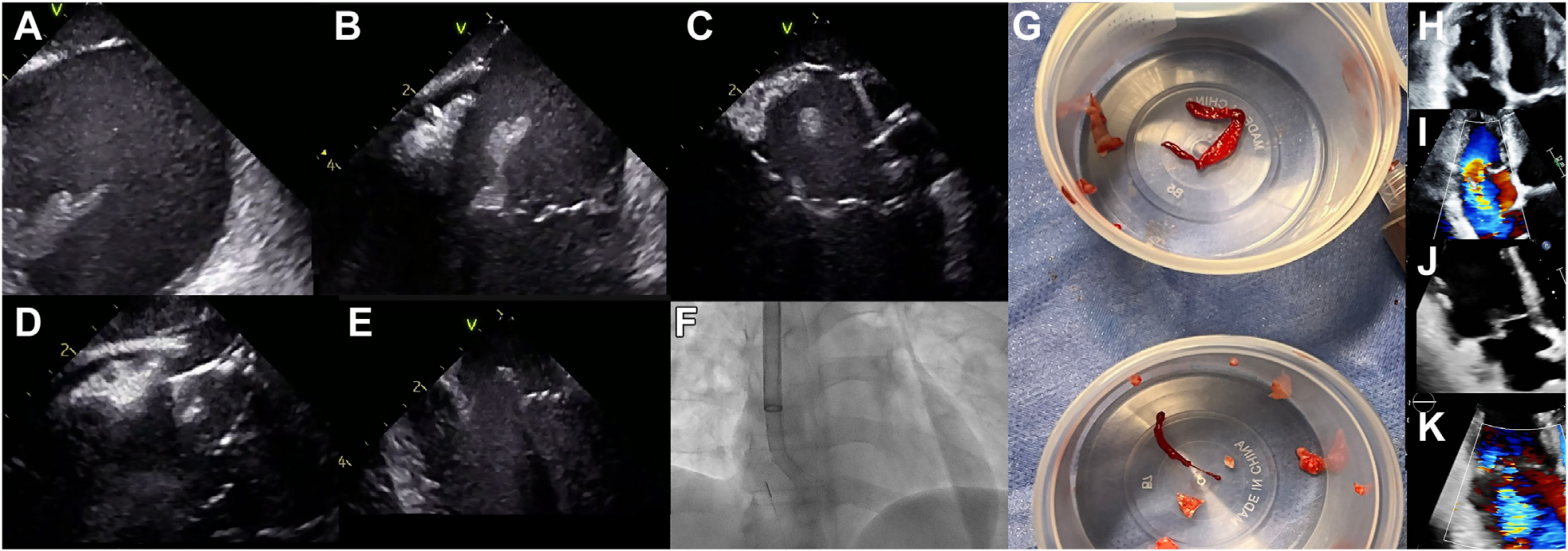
Intracardiac echocardiography-guided percutaneous aspiration of tricuspid vegetations. (A, B) Vegetation on the tricuspid valve. (C) Engaging with the cannula. (D) The vegetation is seen inside the funnel-shaped tip. (E) The leaflet of the tricuspid valve undamaged and free of vegetations. (F) An AlphaVac (AngioDynamics) cannula 26F sheath from the internal jugular vein to the right atrium and an intracardiac echocardiography catheter from the common femoral vein. (G) The aspirated material. (H) Vegetation on the tricuspid valve before aspiration. (I) Severe tricuspid regurgitation before aspiration. (J) The tricuspid valve after aspiration. (K) Moderate tricuspid regurgitation after aspiration.
